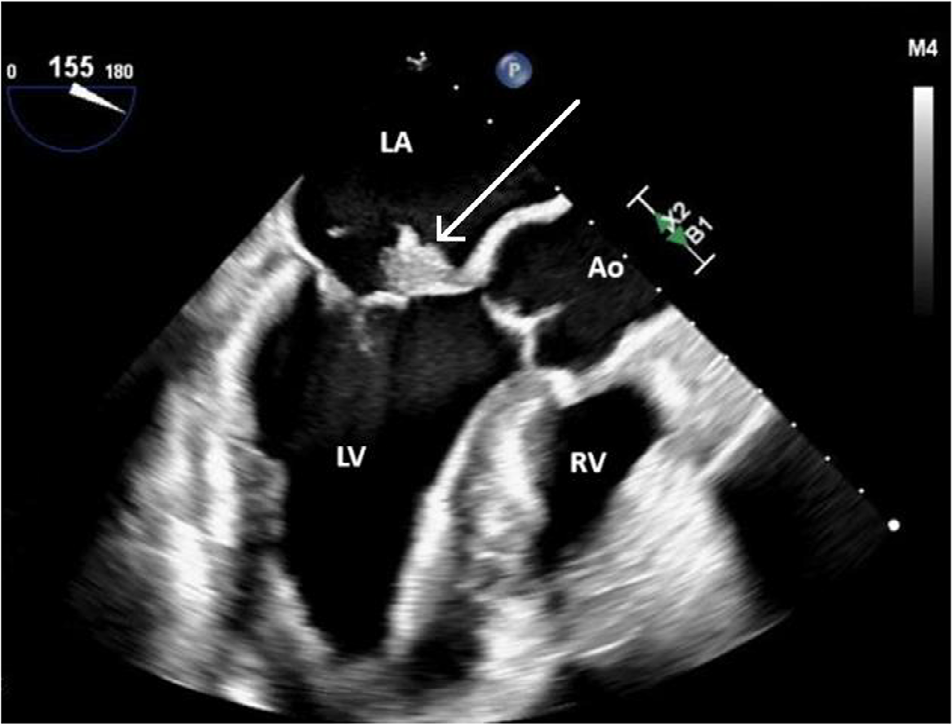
Transesophageal echocardiogram demonstrating a large, echodense vegetation (white arrow) attached to the anterior leaflet of the mitral valve. Ao, proximal ascending aorta; LA, left atrium; LV, left ventricle; RV, right ventricle.
